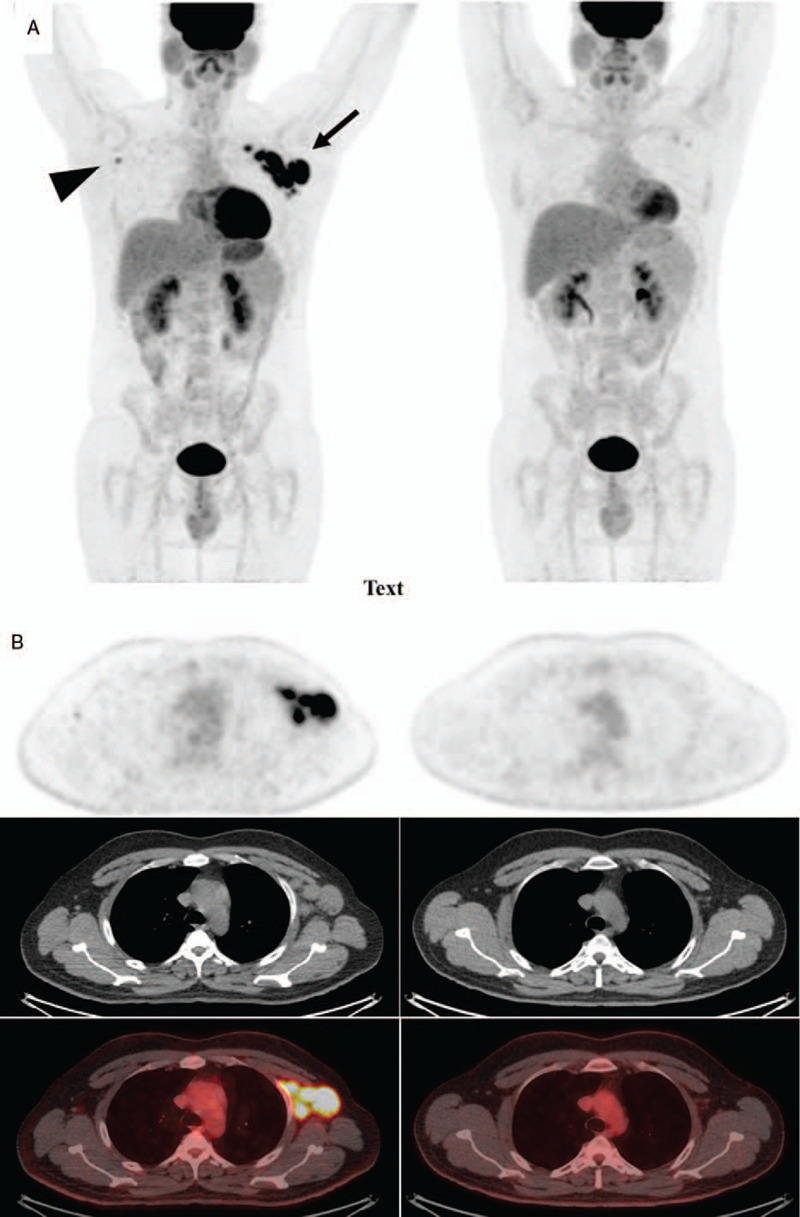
^18^F-FDG PET/CT scans on (left) and 2 months off dasatinib (right). A) Maximum intensity projection images. Left, intense tracer uptake (SUVmax 16.7) is observed in grossly enlarged left axillar and subclavian lymph nodes (arrow) and moderate (SUVmax 4.2) in a small right axillar lymph node (arrowhead). Right, almost complete metabolic normalization of the left axillar lymph nodes with only mild metabolic activity seen in a pair of subclavian lymph nodes and complete normalization of the right axillar lymph node. B) Axial sections at the level of axilla. Upper row, PET sections; middle row, CT sections; lower row, PET/CT sections.
